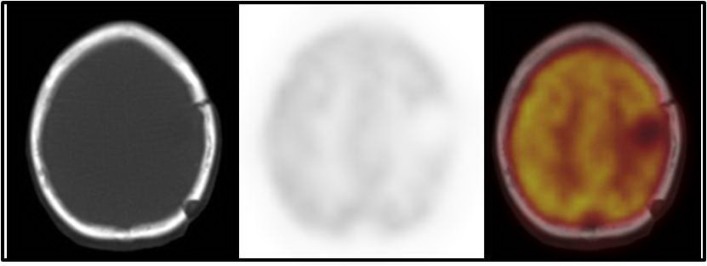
Chest radiography showing a large mass lesion in the upper lobe of the left lung
